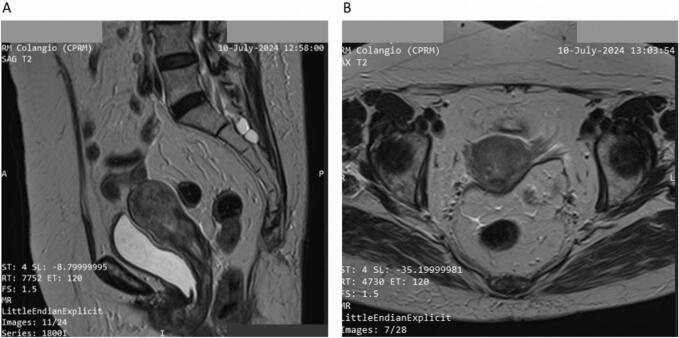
A. Sagittal T2-weighted MRI shows a tumor enlarging the endometrial cavity and protruding into the cervical canal. 3B – Axial T2-weighted MRI demonstrates invasion of the tumor into the outer myometrial wall.
